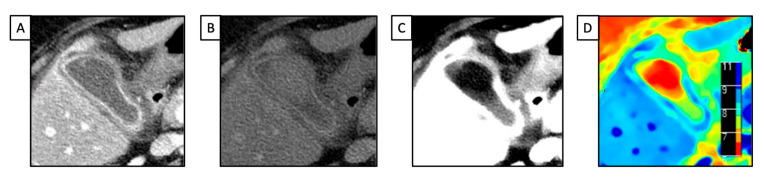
A 42 y-o patient with right upper pain underwent abdominal CT with a final diagnosis of cholecystitis. (A) Conventional CT image acquired after intravenous contrast media injection shows diffuse thickening of the gallbladder wall, without evidence of any calcific stone; (B) low mono-energetic map shows a hypoattenuating round stone, due to cholesteric composition; (C) opposite that, the high mono-energetic map demonstrates the hyperattenuating mass consistent with the cholesteric gallstone; (D) the Z-effective map allows us to better define the different structures of the images via the different atomic values of the gallbladder: blue (contrast agent), red (lipid content), and green (fluid).
